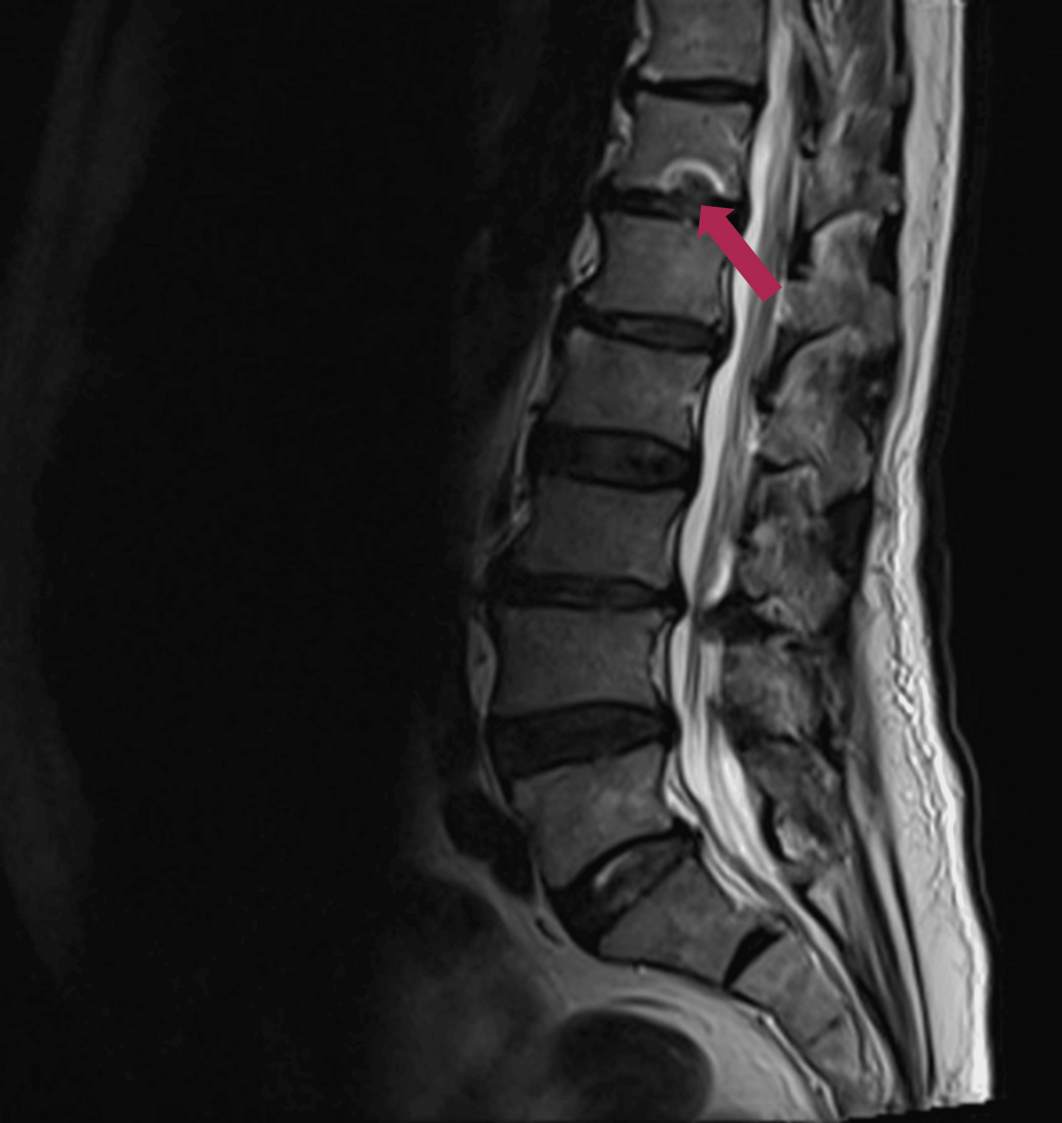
MRI of the lumbar spine demonstrating L4 to L5 facet joint septic arthritis with a small paraspinal collection Bilateral L4 to L5 facet joint septic arthritis with adjacent paraspinal collection (11.5 × 11.2 mm) and milder L3 to L4 inflammatory changes. No discitis, epidural abscess, or cauda equina compression.
